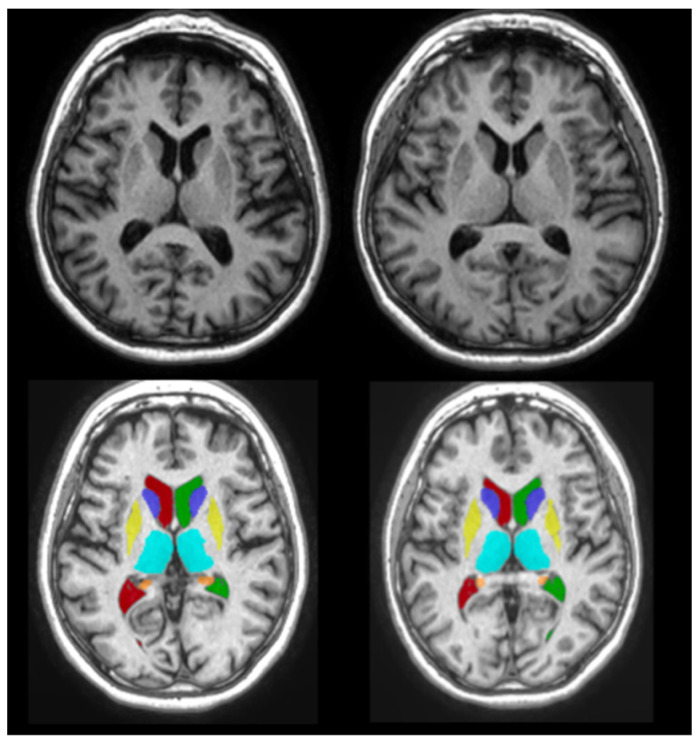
The 3D T1-weighted magnetic resonance images of 69-year-old monozygotic twins taken at the Medical Imaging Centre, Semmelweis University. The images were segmented using volBrain. The subcortical structures were delineated and their volumes were calculated.
